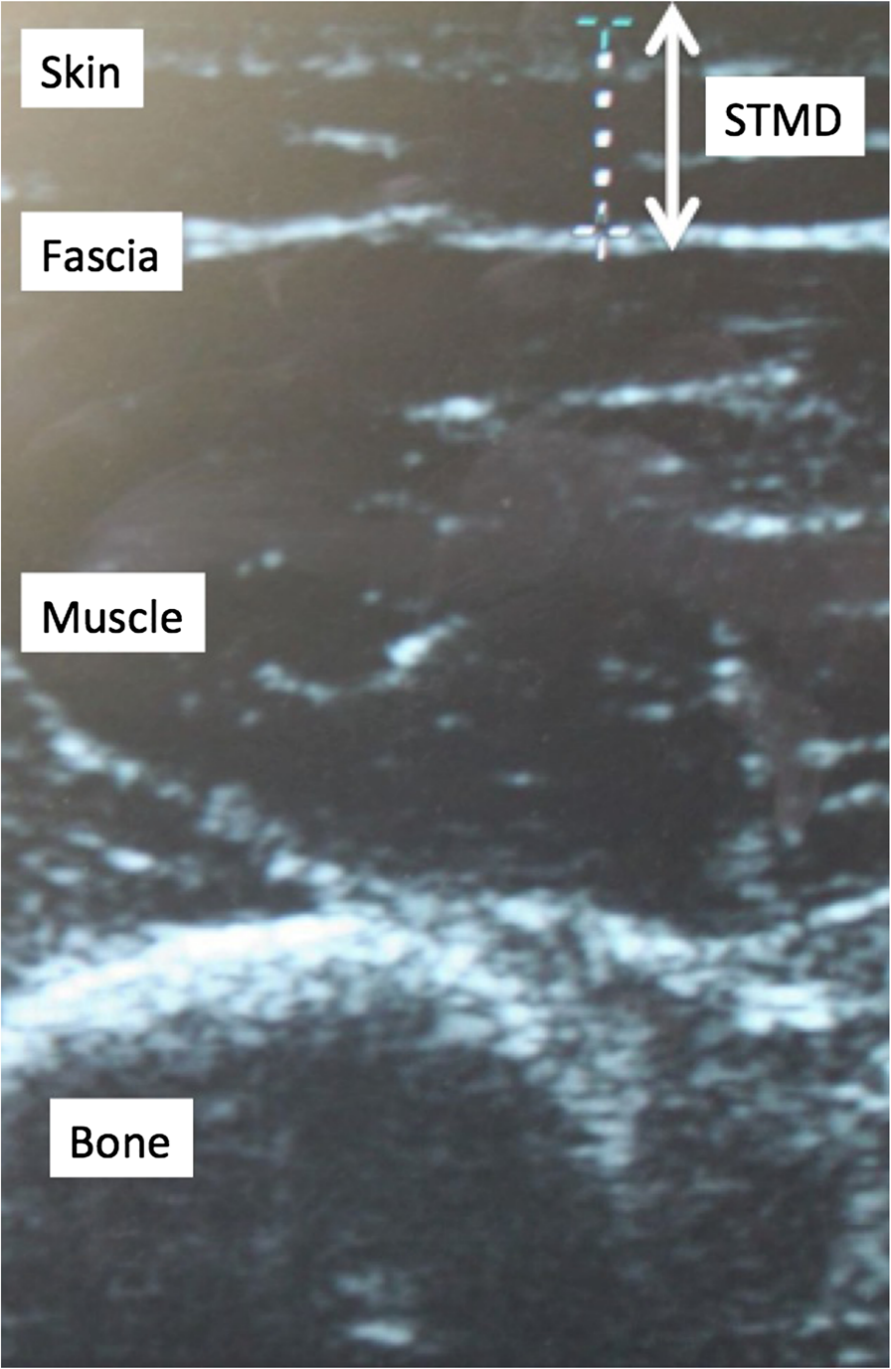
Image of ultrasound showing skin-to-muscle depth (STMD).
